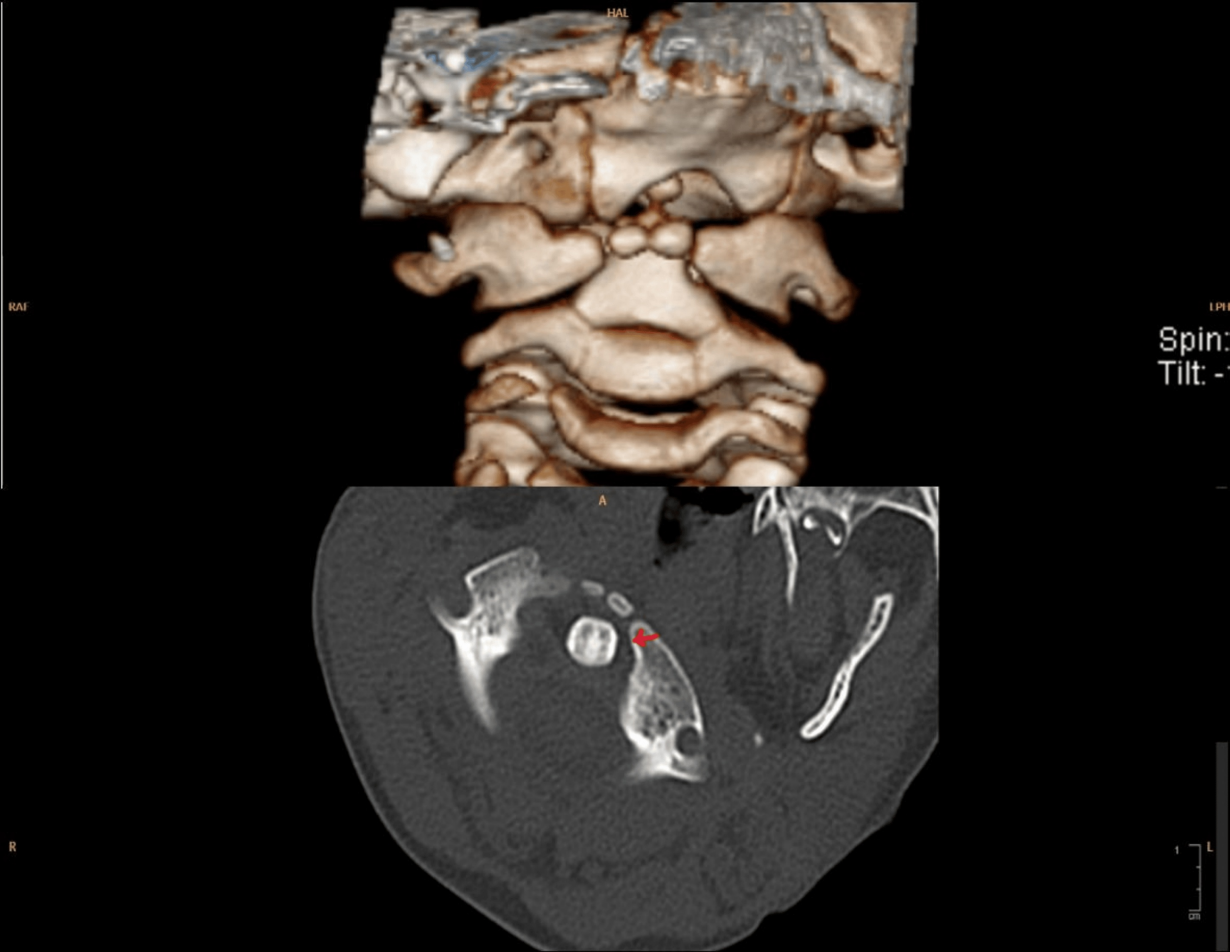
Atlantoaxial subluxation showing in the 3-Dimensional and a axial view of a CT cervical spine A: 3-dimensional view; B: the axial view.
